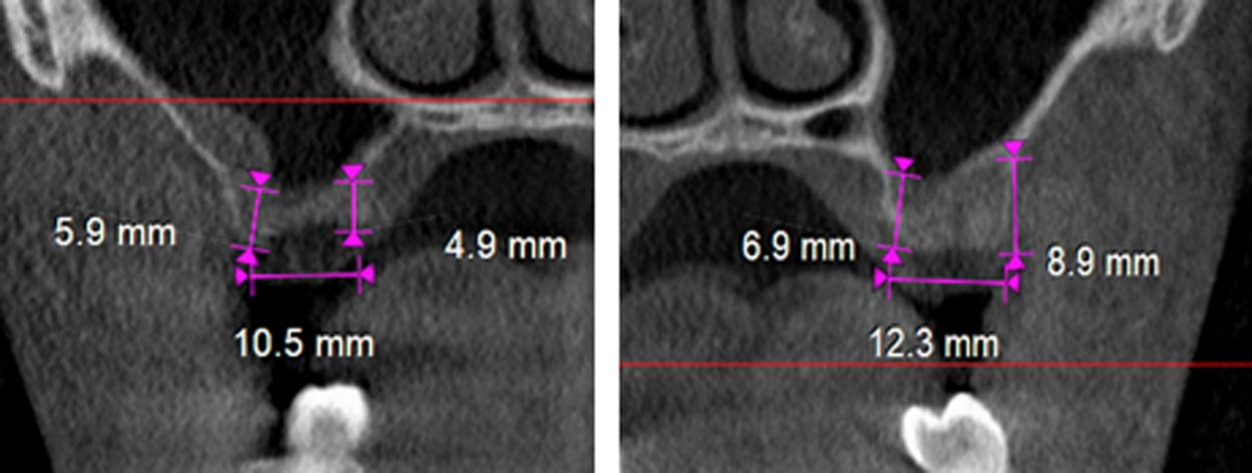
Shows the measurements of alveolar bone dimensions using CBCT scan after the surgical procedure (somatropin was applied in the socket of upper left molar.
